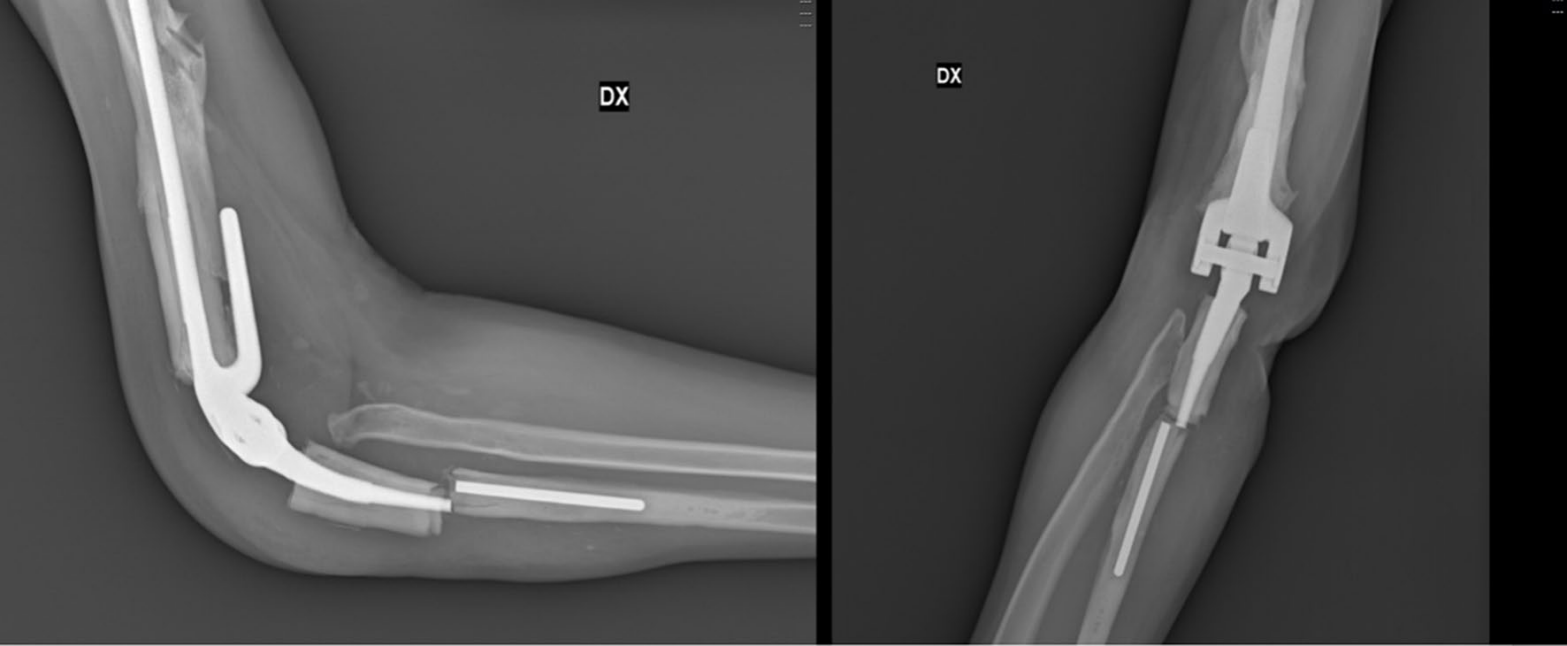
X-rays of a periprosthetic ulnar fracture and Coonrad Morrey stem fracture
